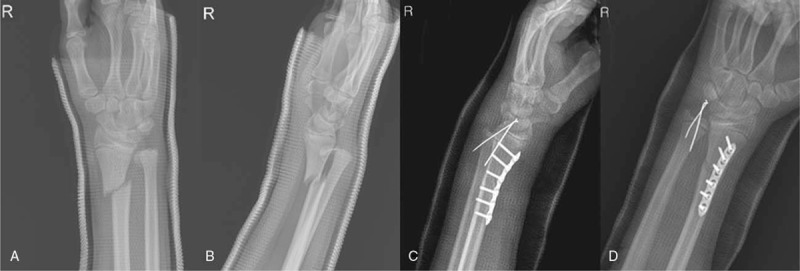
(A, B) Galeazzi equivalent fracture, pronation type. Preoperative AP and lateral views showing volar displacement of radius. (C, D) Postoperative x-rays.
